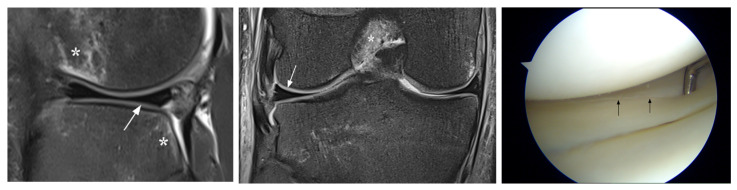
Unavoidable false-negative MRI diagnosis (patient 5). MRI shows normal appearance of the posterior horn of the lateral meniscus (arrows), whereas the arthroscopic image indicates an upper surface tear (arrows). Note bone contusions (*) at the lateral knee compartment due to acute ACL injury.
